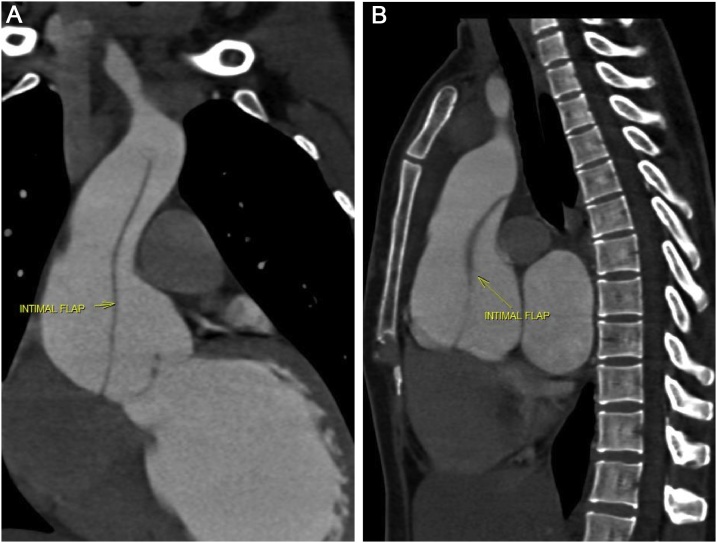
A and B: Coronal and Sagittal Multiplanar Reconstruction (MPR) of the 75% phase of the gated CT Aortogram study showing type A aortic dissection extending to the aortic root.
